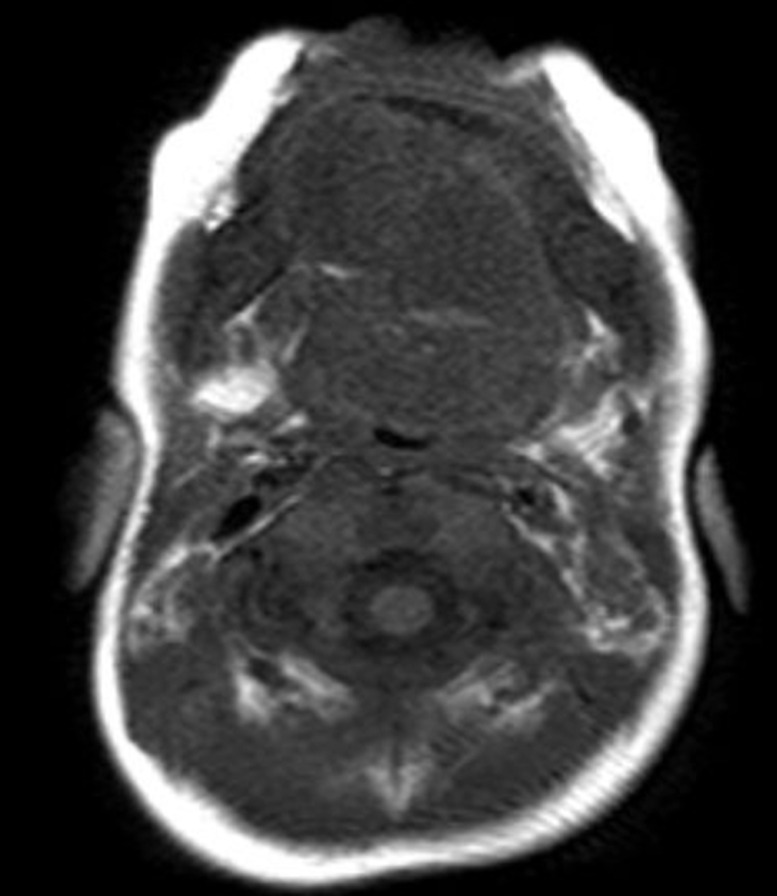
Imaging of 2-month-old female patient with sublingual dermoid cyst. In T2W, MRI showed well-defined, well-circumscribed, homogenous cystic lesions adjacent to each other, involving the right and midline of the floor of the mouth. The lesions measured approximately 15 × 14 × 12 mm and 15 × 13 × 11 mm.
